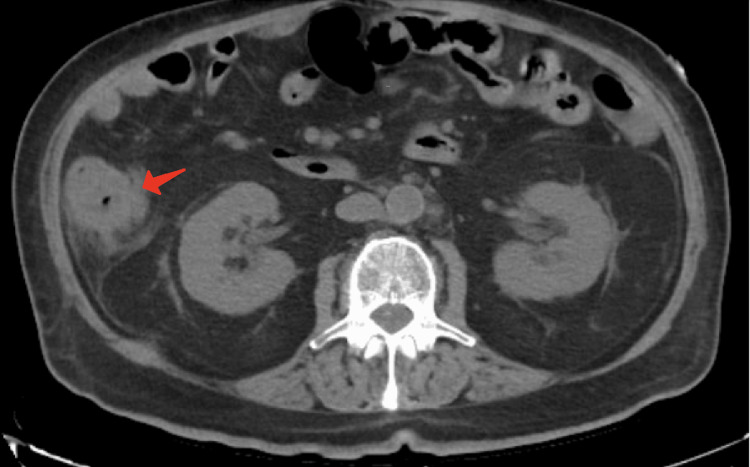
CT scan of the abdomen and pelvis showing inflammation of the cecum and ascending colon, findings consistent with typhlitis
